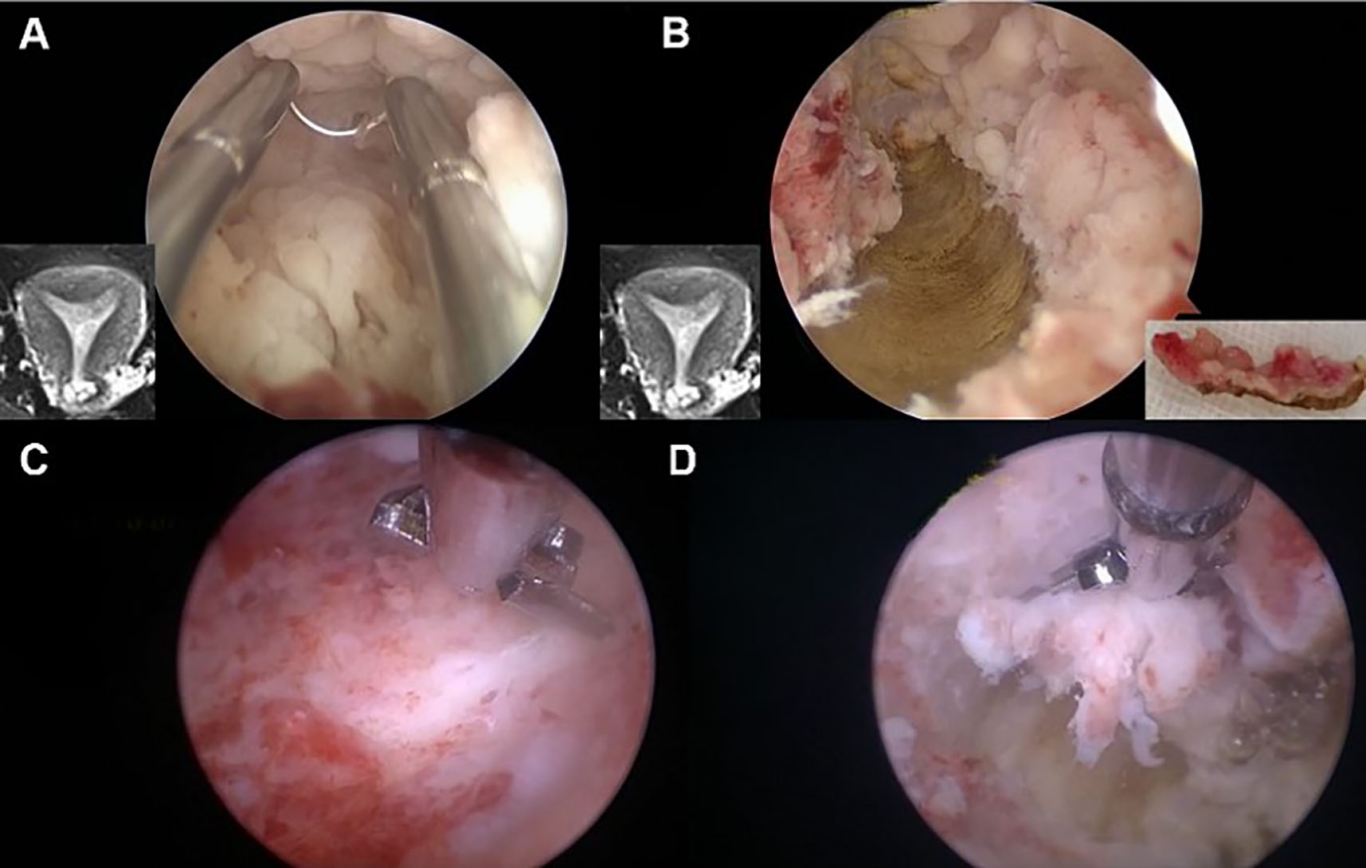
Hysteroscopic one-step resection in early EC patients. (A) The lesions suspected of muscular involvement were accurately located under hysteroscopy according to MRI. (B) During electrosurgical resection, “one continuous resection” was applied to resect the myometrium, with the endometrium connected to the myometrium. (C, D) The residual lesions were removed with scissors to reduce electrical damage to the endometrium and prevent uterine adhesions.
